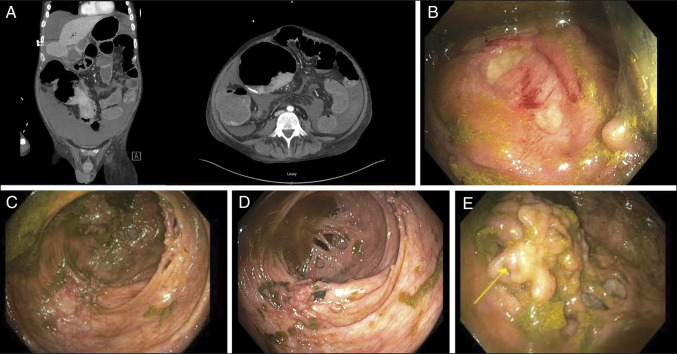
(A) Computed tomography of the abdomen demonstrating diffuse bowel distention with narrowing at the colonic anastomosis. (B) Colonoscopic view of circumfrential inflammatory psuedopolyps. (C) Advancement with a pediatric colonoscope demonstrating multilobulated mucosal projections and extensive mucosal bridging. (D) Cecal examination with additional mucosal bridges forming obstructive intraluminal tracts. (E) Ileocacal valve with pseudopolypoid inflammatory change and adjacent mucosal bridging.
